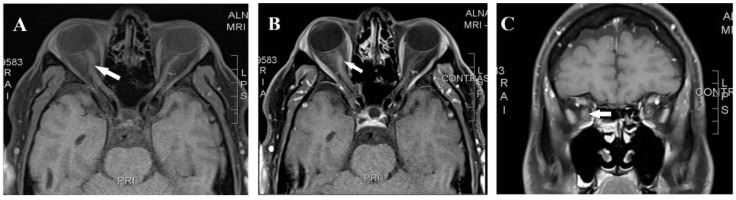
Magnetic resonance imaging axial T1 pre-contrast (A), axial T1 post-contrast (B), and coronal T1 post-contrast (C) showed right-optic-nerve mild enhancement and enlargement (arrow).
